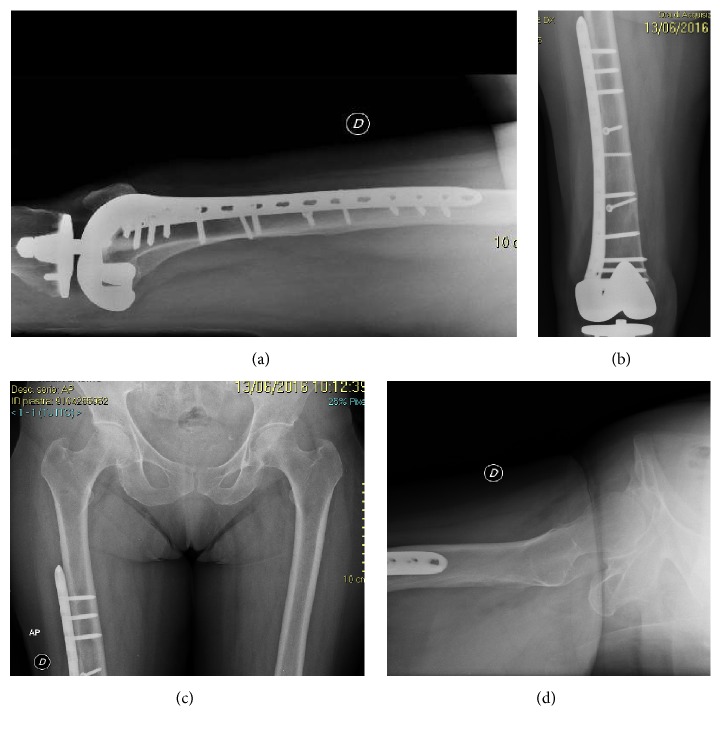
X-ray examination at 2-year follow-up.
